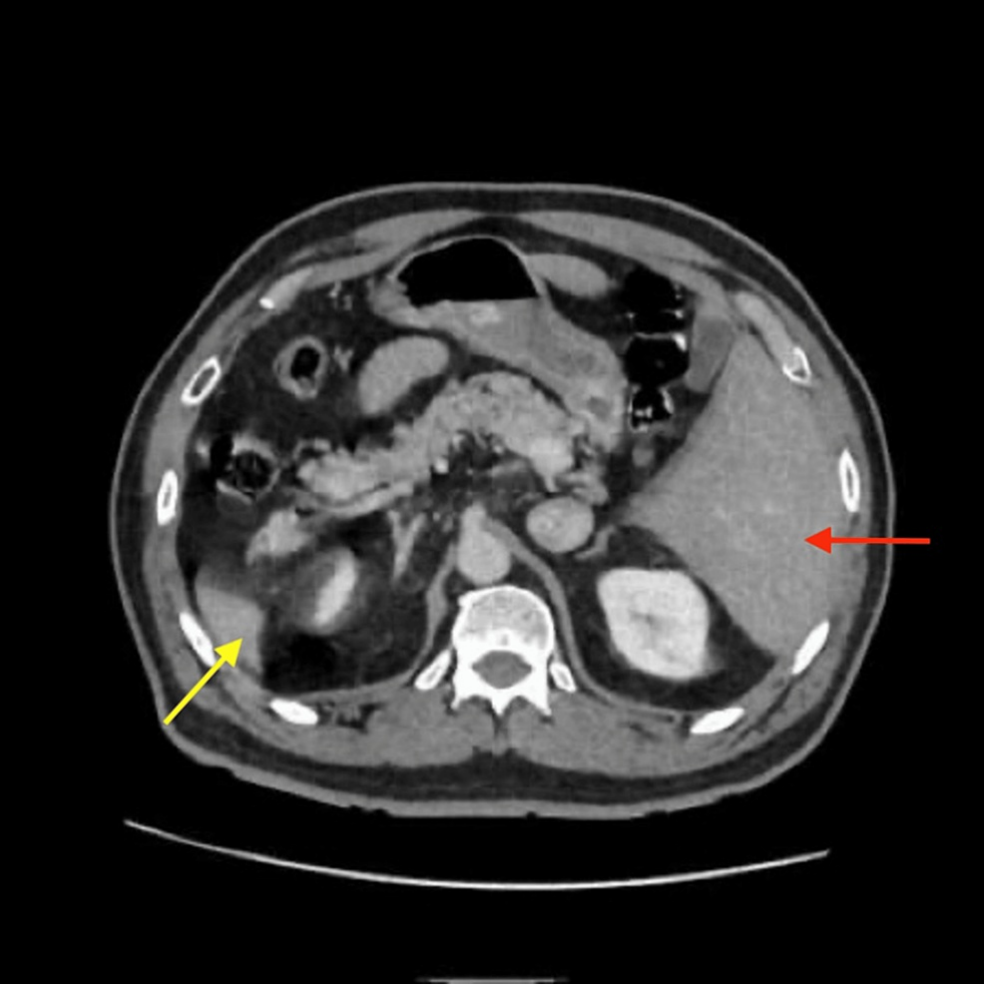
CT scan of the abdomen showing left-sided liver (red arrow) and right-sided spleen (yellow arrow)
